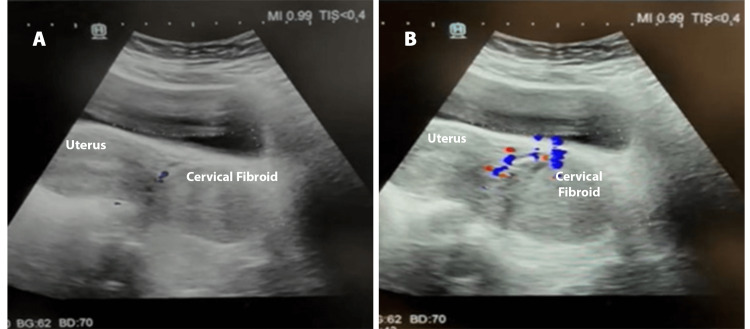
Ultrasound of the pelvis with Doppler. (A) A large cervical fibroid measuring 14.5 × 10.5 cm. (B) Cervical fibroid showing marked vascularity.
